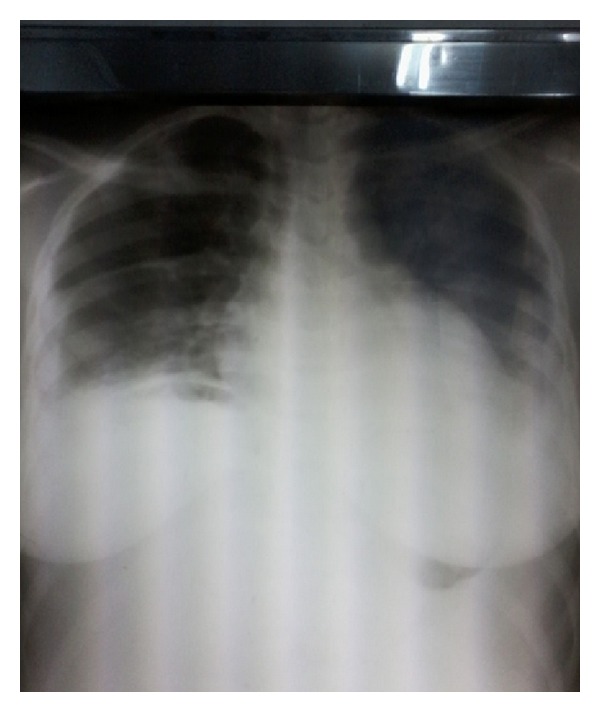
Chest radiograph showing a bilateral hydrothorax.
